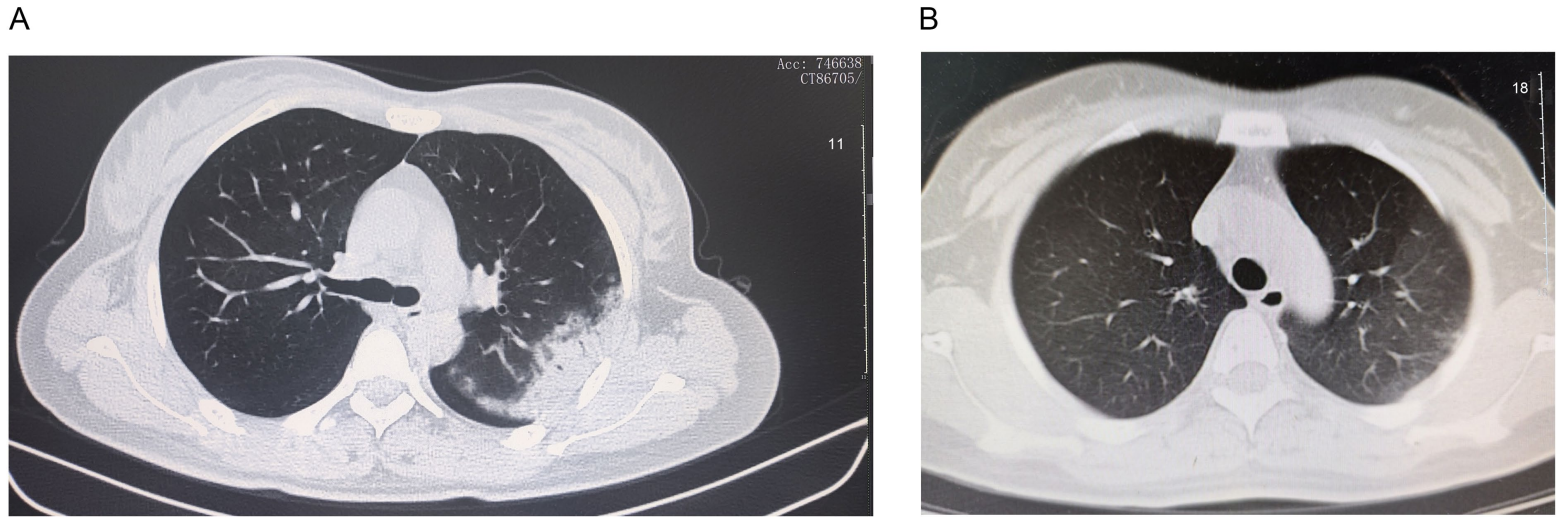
Clinical and radiological findings in the index patient with psittacosis. (A) Chest CT obtained on day 4 after symptom onset (July 24, 2024), showing extensive consolidation with surrounding ground-glass opacity in the left upper lobe. (B) Follow-up CT on day 11 (August 1, 2024) after initiation of doxycycline therapy, demonstrating significant resolution of the consolidation.
